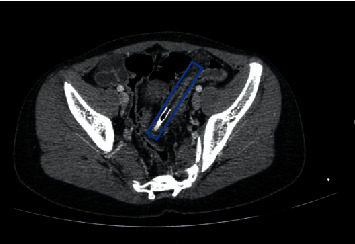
Computed tomography imaging of a 14-year-old boy with evidence of a foreign body (paintbrush) in the pelvic cavity, anterior to sigmoid bowel loops (marked with blue outline).
